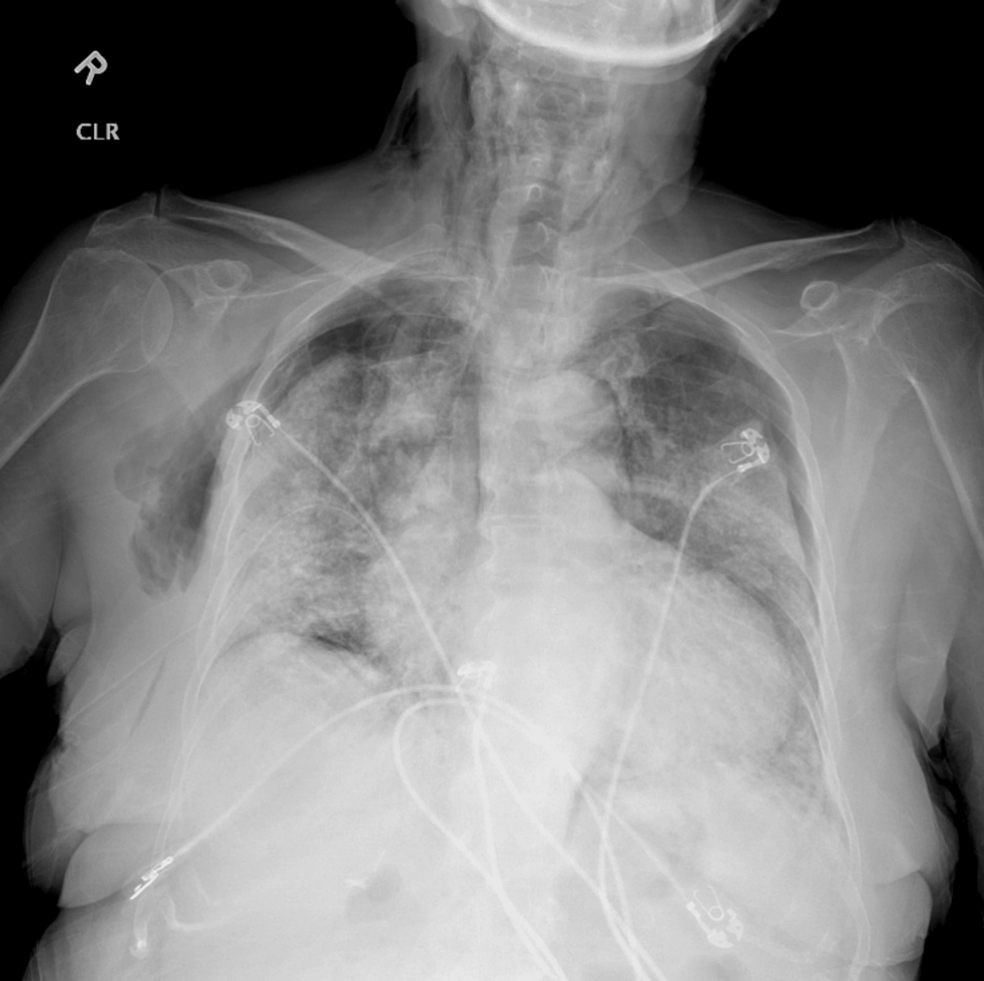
Chest radiograph showing bilateral pneumothorax, pneumomediastinum, and extensive subcutaneous emphysema in the neck and chest wall.
